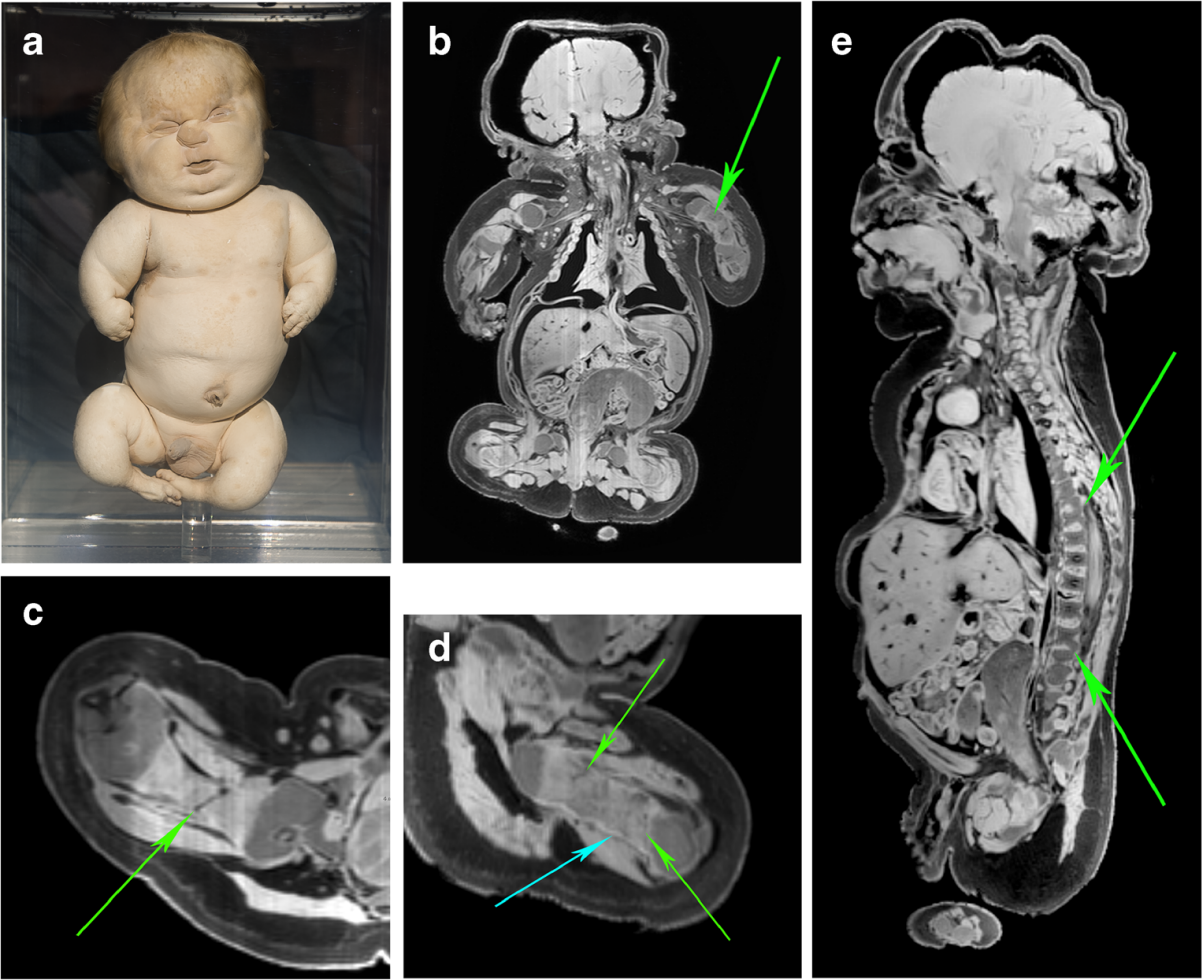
a Photograph of the full-term fetus of case 2 demonstrating a characteristic facial appearance, protruding abdomen and severely bowed extremities. b Coronal T1-weighted MR image showed an aberrant and irregular humerus (*green arrow*). Note the severely shrunken lungs insight the hypoplastic thorax; interpreted as shrinkage of the lungs due to post-mortem artefacts and formalin fixation. However, the presence of lung hypoplasia cannot be ruled out. c Transverse T1-weighted MR image of the broadened and shortened right femoral bone which showed a fracture (*green arrow*). d Transverse T1-weighted MR image of the severely shortened and aberrant left femoral bone which showed multiple fractures (*green arrows*) and irregular cortical bone (*turquoise arrow*). e Sagittal T1-weighted MR image showed multiple fractures in the vertebral column (*green arrows*). Note the distorted calvarium due to limited mineralisation and the shrunken brain due to the formalin fixation
